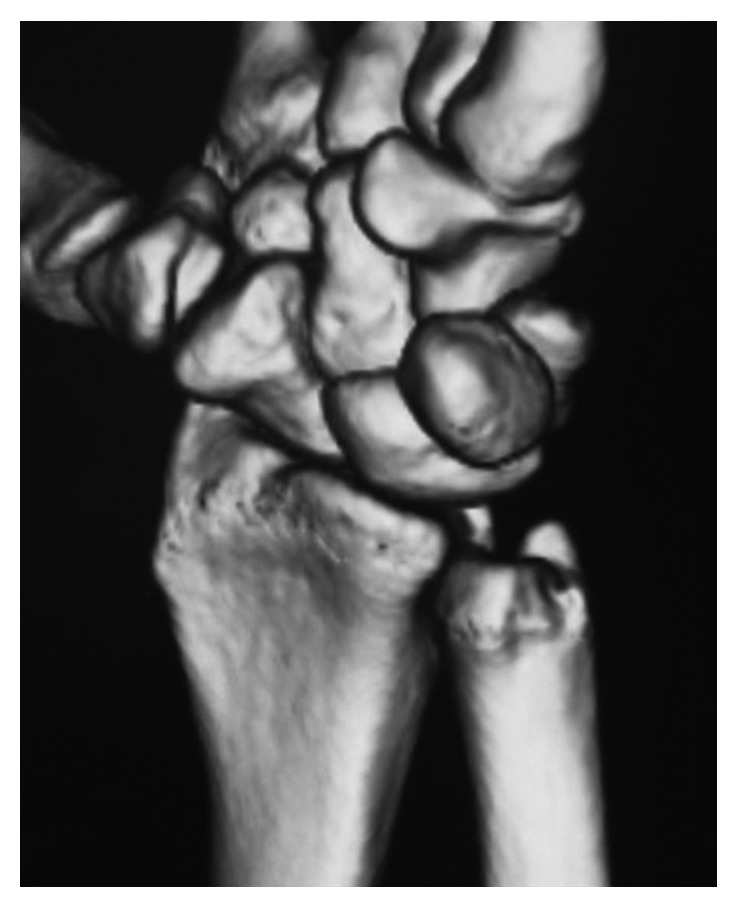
3D reconstruction of CT at 18 months postoperatively. It seemed the ulnar head had remodeled.
